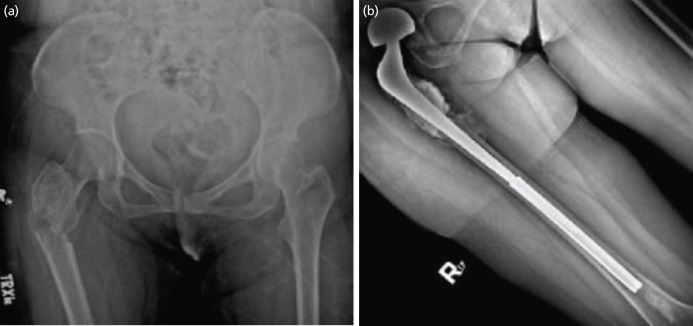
(a) Injury radiograph of the pelvis in AP view, with an intertrochanteric fracture in the right proximal femur and note of a lytic lesion in the area of the per trochanteric area. Bone scan later on revealed presence of metastatic lesions in the diaphysis of the right femur. (b) Post-operative radiograph of the femur in AP view at latest follow-up at one year and three months, with no signs of dislocation, recurrence or implant failure observed.
